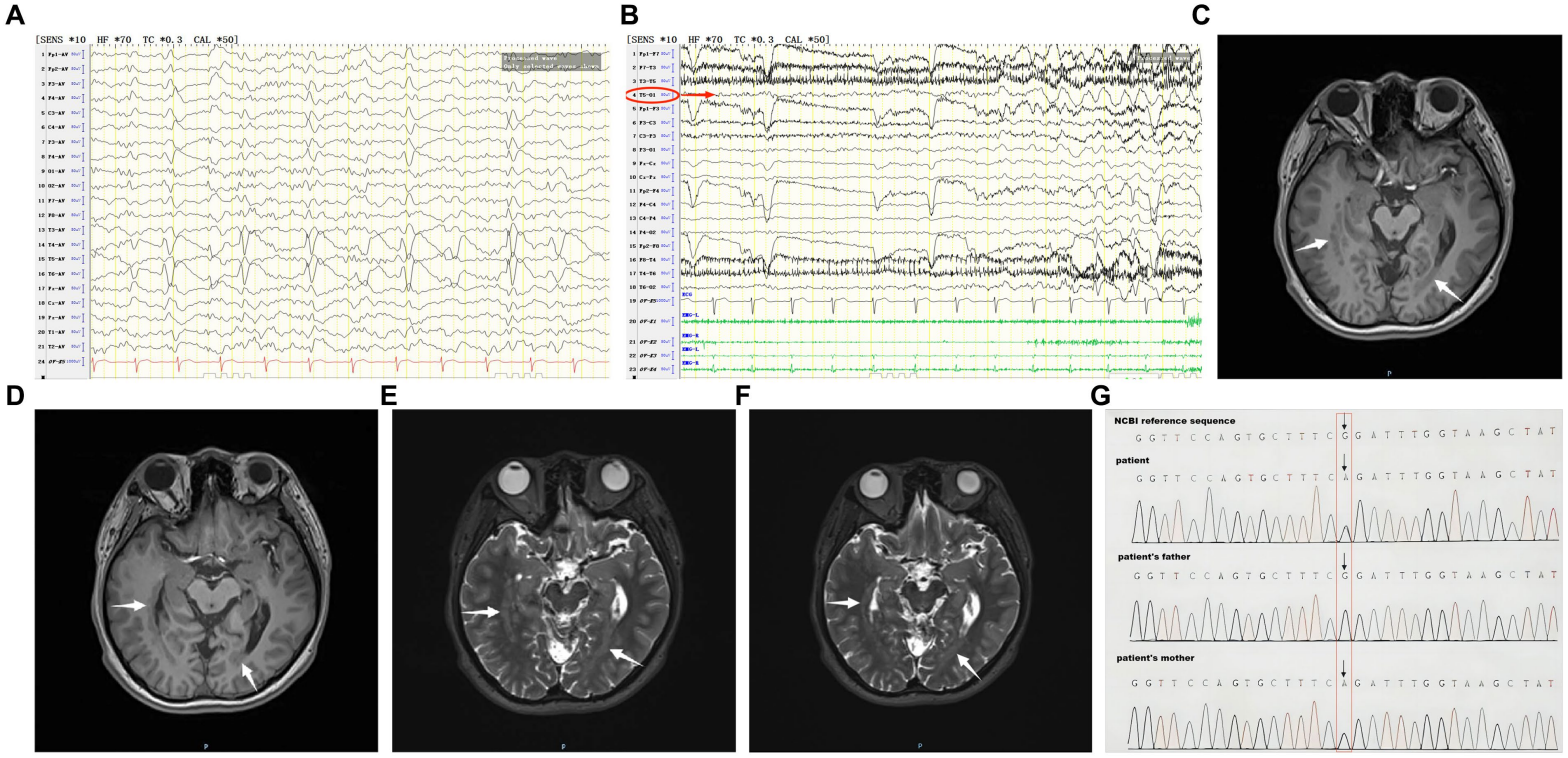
(A) The patient’s interictal period EEG. (B) The patient’s ictal period EEG. (C–F) The patient’s MRI before coagulation. (G) Whole-exome sequencing of monogenic disease.
